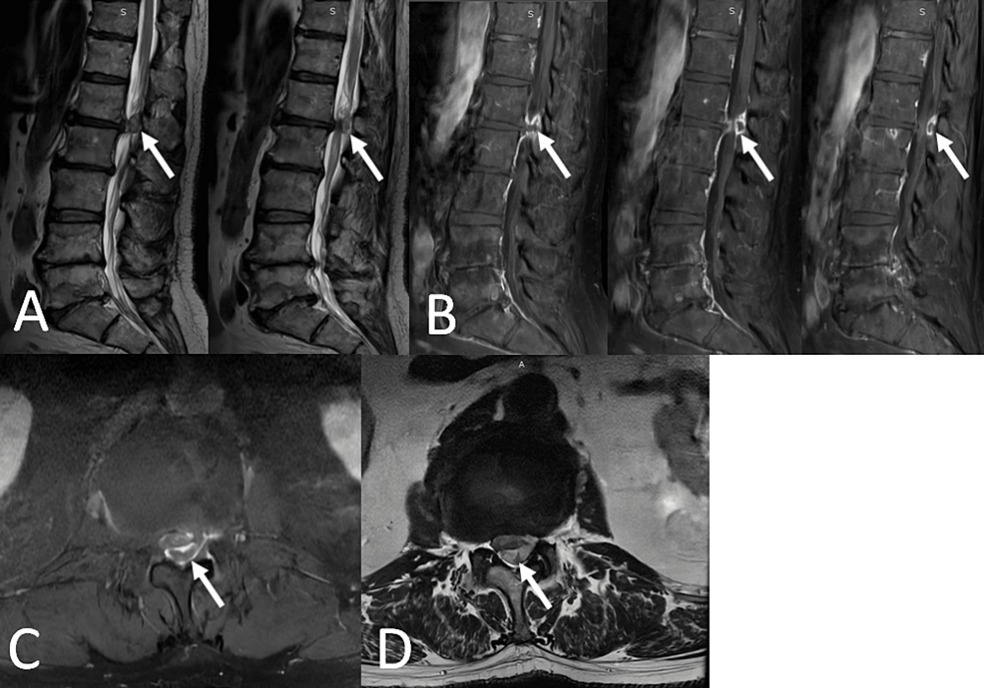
(A) Sagittal T2-weighted images demonstrate a large mass in the L1-L2 epidural space. (B) Gadolinium-enhanced sagittal T1-weighted images show a peripherally enhancing lesion in the L1-L2 epidural space that is elongating posterolaterally. (C) Gadolinium-enhanced axial T1-weighted images demonstrate a peripherally enhancing lesion (arrow) located in the anterior-to-posterior epidural space at the L1–L2 level. (D) The same lesion is seen in the T2-weighted images.
